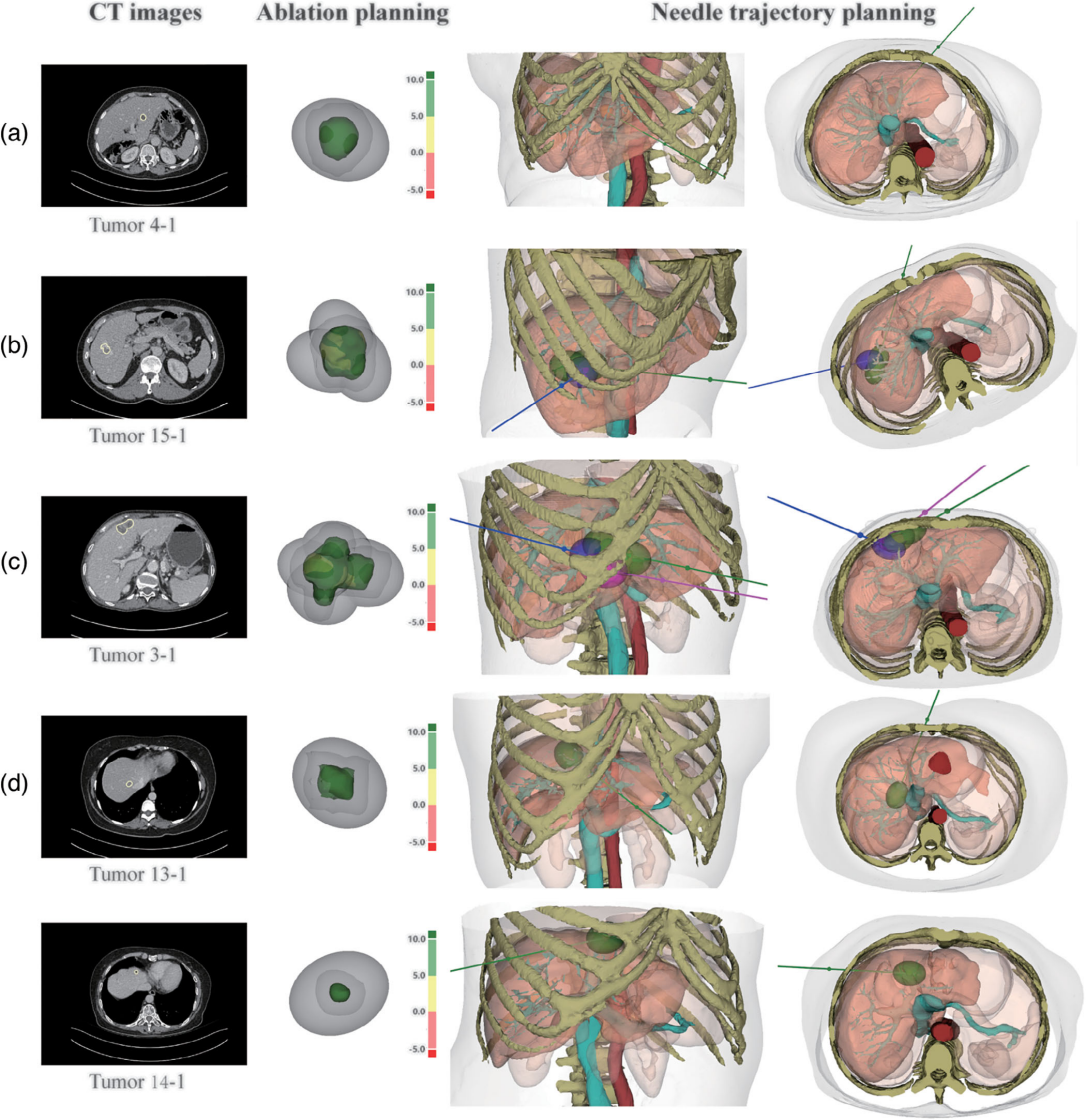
Visualization of the ablation and needle trajectory planning results. (a) The single‐needle planning results (Tumor 4‐1). (b) The multi‐needle planning results include a pull‐back trajectory represented by a green line (Tumor 15‐1). (c) The planning results for the largest tumor (Tumor 3‐1, with a tumor size of 35.5 mm) in our Test Dataset, where the pull‐back trajectory is represented by a green line. (d) The planning results for the tumor located at the hepatic dome (Tumor 13‐1 & 14‐1). Each column demonstrates the axial CT images including the target tumor contoured by the white circle (first column), the ablation planning results (second column) where the color distance map shows the distance between the tumor and ablation zones, and the needle trajectory planning results in 3D views (third and fourth columns).
